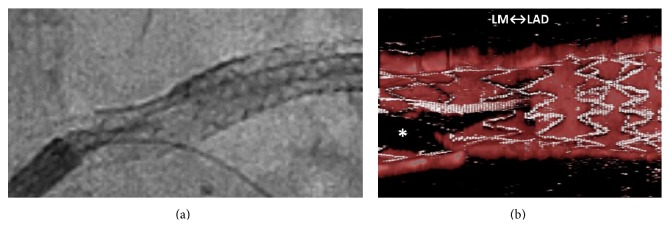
Third rewiring. (a) X-ray fluorography, (b) intraprocedural instant stent-accentuated three-dimensional optical coherence tomography showing the rewiring through the larger distal cell. LM: left main coronary artery, LAD: left anterior descending artery, and *∗*: guide wire shadow artifact.
